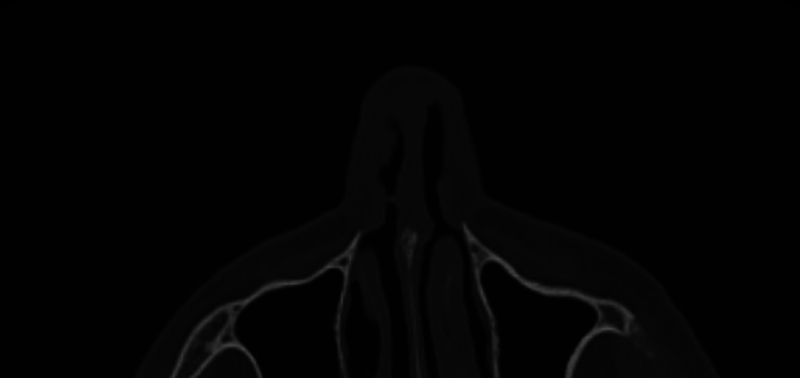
Preoperative CT: squamous cell carcinoma of the right nasal cavity with involvement of the right nasal valve.
